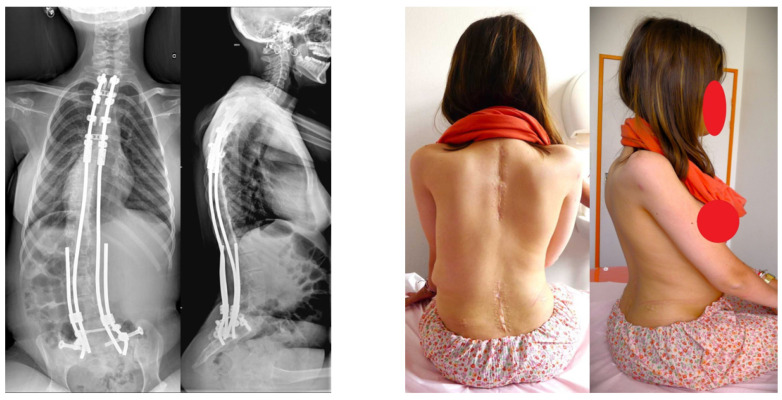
Postoperative X-rays and clinical pictures of the same patient, after minimally invasive fusionless surgery.
